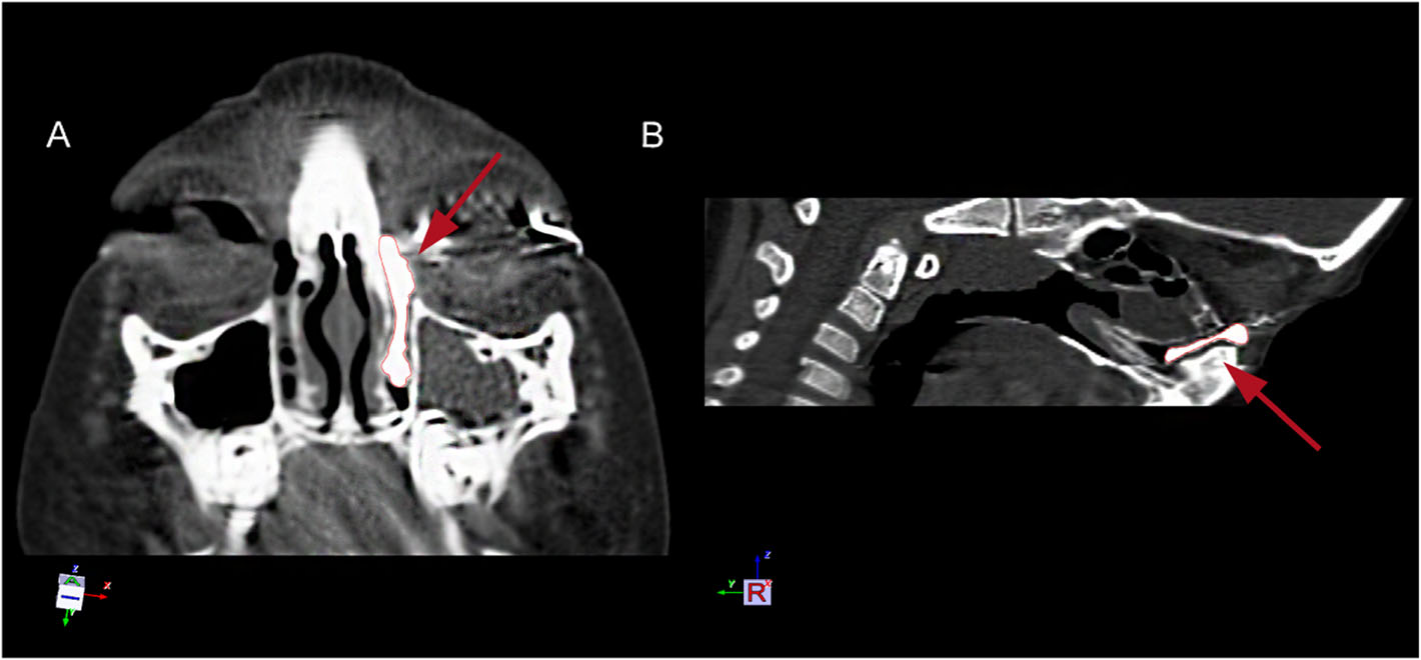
CT-DCG of a membranous CNLDO patient. a. An axial image. b. A sagittal image. a and b. The contrast medium was blocked at the end of the nasolacrimal duct, showing the shape of the whole duct. The enlarged Hasner’s valve was shown by the red arrows
